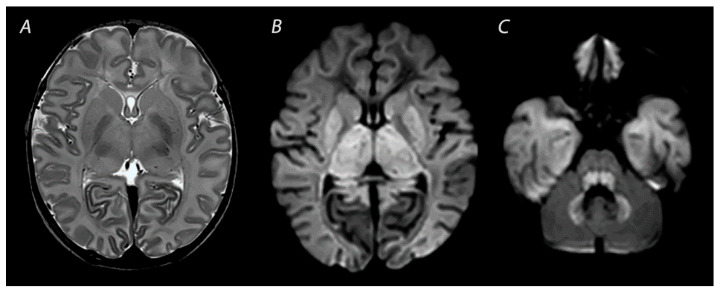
MRI performed on day 3 after birth, showing near total injury with extensive injury in the white matter, BGT, brainstem and cerebellum. Abnormalities are often more subtle on T2 weighted imaging in the first days after the hypoxic-ischemic insult (A) and are best seen using DWI on early MRI (B,C). Except for the dentate nuclei, the signal in the cerebellum is not increased and therefore the term ‘the white brain’ was coined. The infant died after redirection of care.
